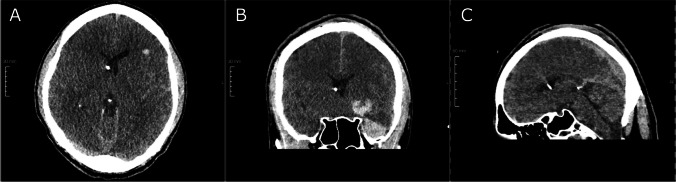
Representative postoperative cranial CT demonstrating an optimally placed EVD. Axial (A), coronal (B), and sagittal (C) cranial CT slice of a patient with narrow ventricles and intracerebral hemorrhage (ICH) at the level of the EVD tip
